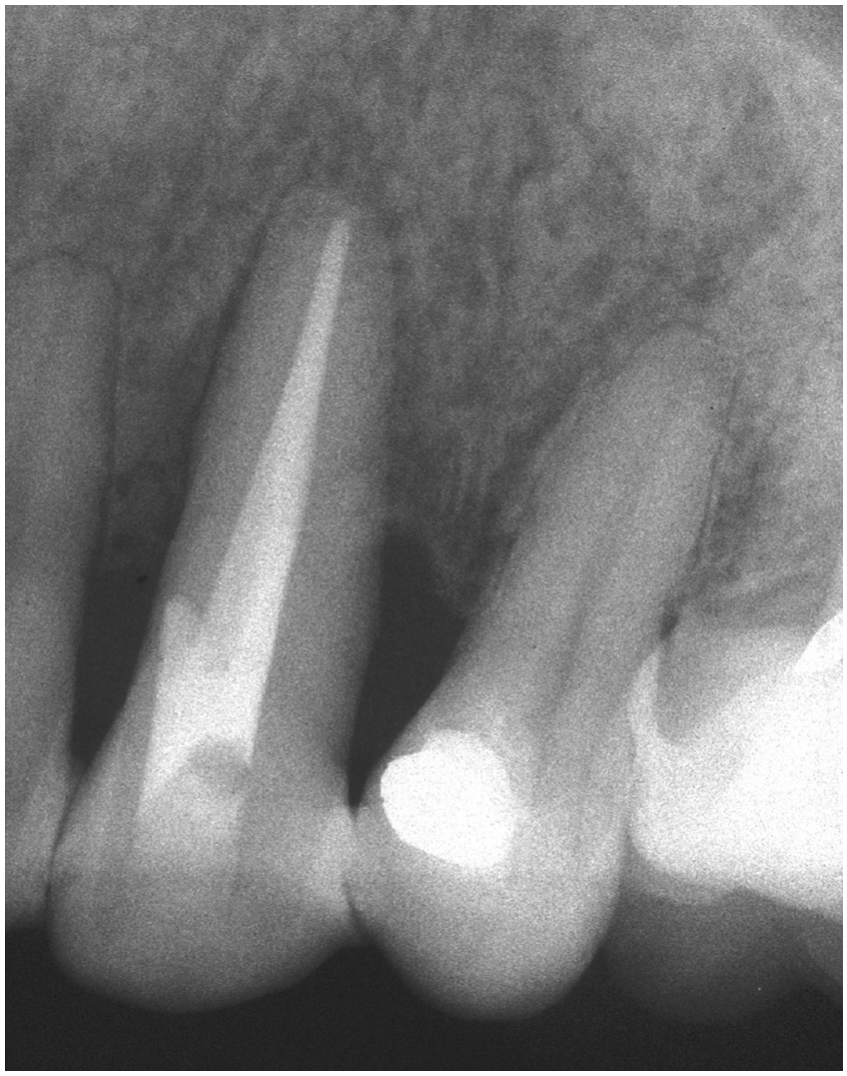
Radiograph shows a slight lateral radiolucency at 2 years follow-up.
